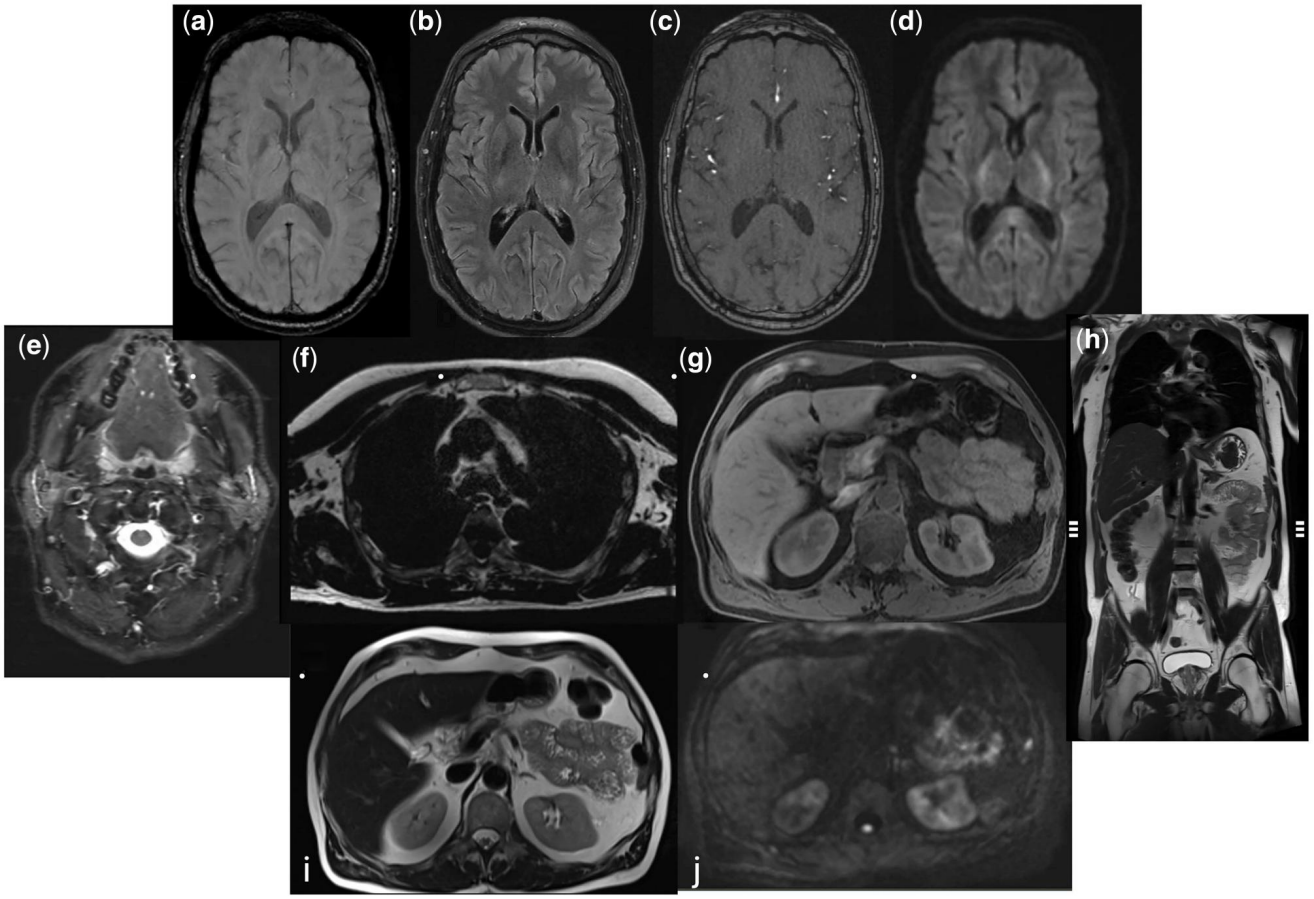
Representative MR Sequences of the Screening Whole Body MRI Protocol. Axial Brain Susceptibility Weighted Imaging (a), Fluid Attenuated Inversion Recovering (FLAIR) (b), Time of Flight MR Angiogram (c), High b-value (b=800) diffusion weighted imaging (d), axial Short Taue Inversion Recovery (STIR) through the neck (e), axial T1-weighted isotropic gradient recalled echo (GRE) with Dixon through the chest (f), whole body axial T1-weighted GRE with Dixon (g), coronal T2-weighted Half Fourier Single Shot Turbo Spin Echo (HASTE) through the abdomen and pelvis (h), and axial whole body T2-weighted HASTE (i), and diffusion weighted imaging (b=800) (j).
